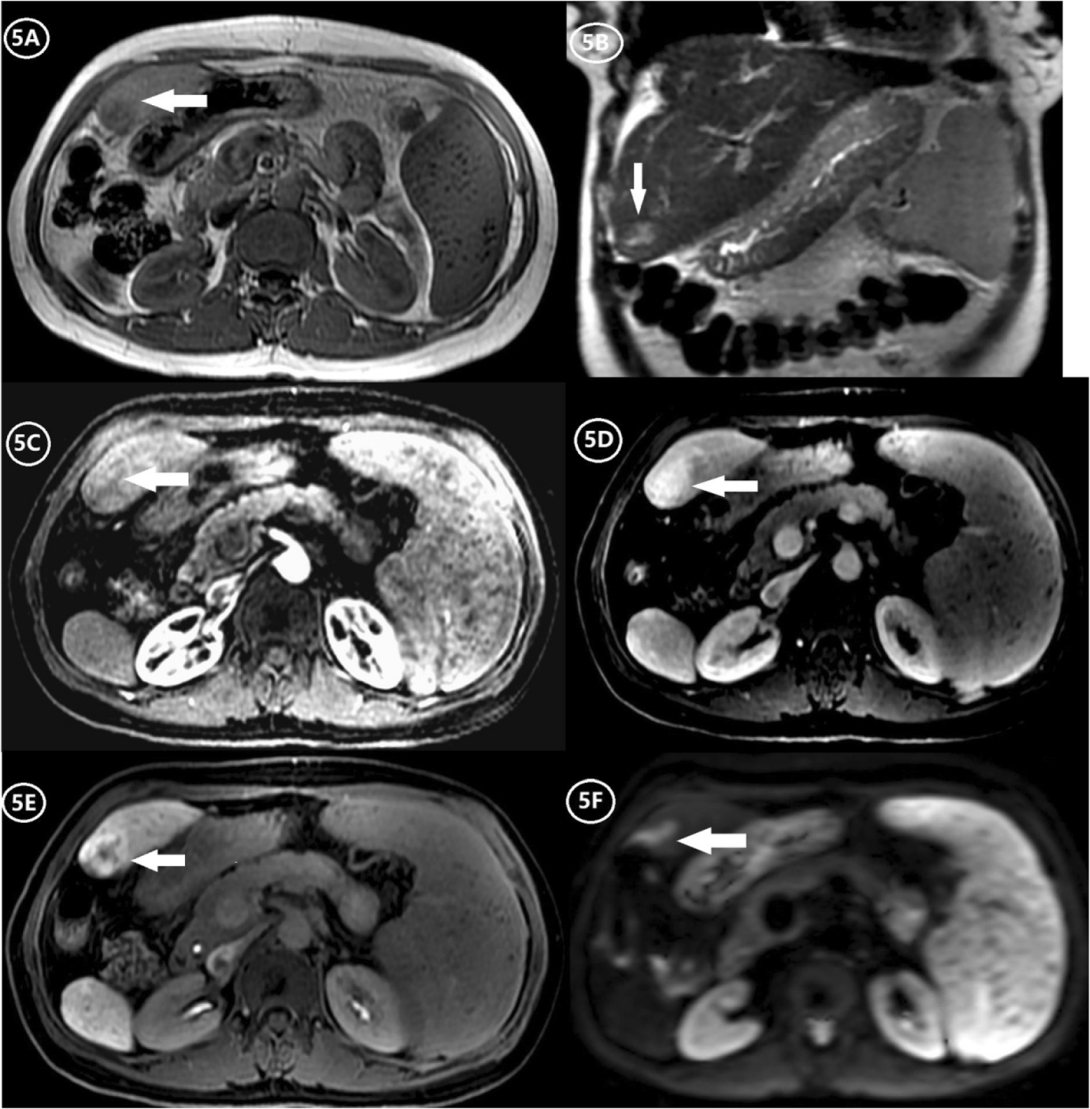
A 48-year-old woman with focal SOS (arrows) developed by chemotherapy after hepatectomy. a, b A nodular lesion in liver segment III with low-signal intensity on the T1-weighted image (T1WI) and high-signal intensity on the T2-weighted image (T2WI). c Arterial phase contrast-enhanced MRI scan shows a nodular lesion in liver segment III with mild peripheral enhancement. d Portal phase contrast-enhanced MRI scan shows the nodular lesion with apparent, uniform, and persistent enhancement. e HBP of gadoxetic acid-enhanced MRI shows the nodular lesion with a low-signal intensity at the center and high-signal intensity around it, which presents as “focal nodular hyperplasia” like lesion. f The diffusion-weighted imaging (DWI) shows an apparent limited diffusion
